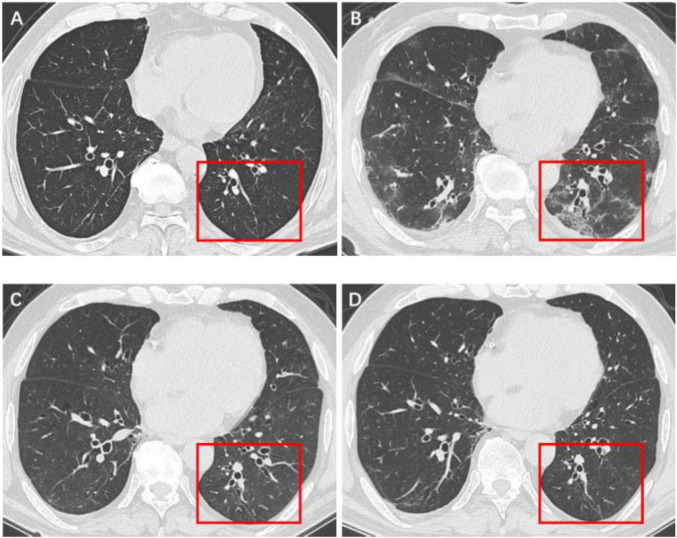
Serial high-resolution noncontrast chest CT in a 61-year-old man with COVID-19 pneumonia. (A) Health screening chest CT 3 months prior to COVID-19 infection showed no abnormalities in the lower lobe of left lung (red box). (B) Initial CT scans obtained on day 6 after the onset of symptoms showed multiple GGOs and reticulation (red box). (C) CT scans obtained on day 173 showed localized residual bronchiolectasis (red box). (D) CT scans obtained on day 375 showed persistence of the bronchiolectasis (red box).
